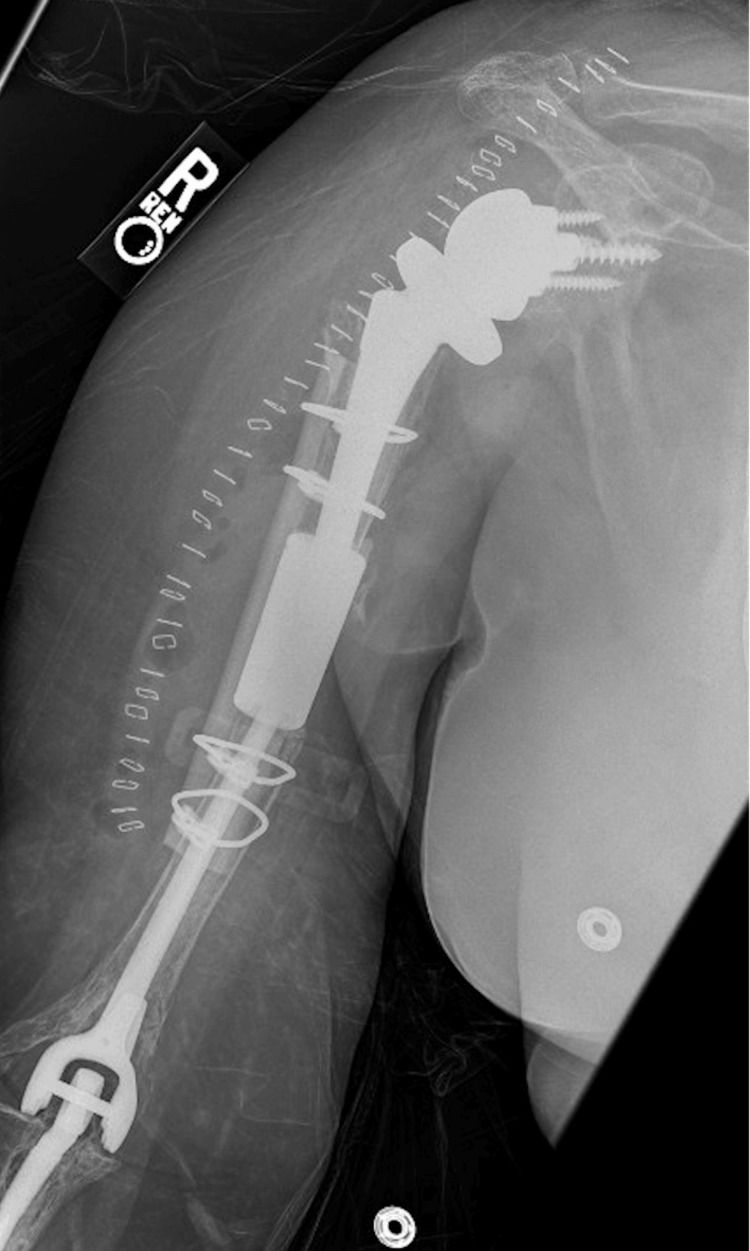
POD0 AP radiograph of the right humerus with allograft repair and RTSA. POD: postoperative day; AP: anteroposterior; RTSA: reverse total shoulder arthroplasty
